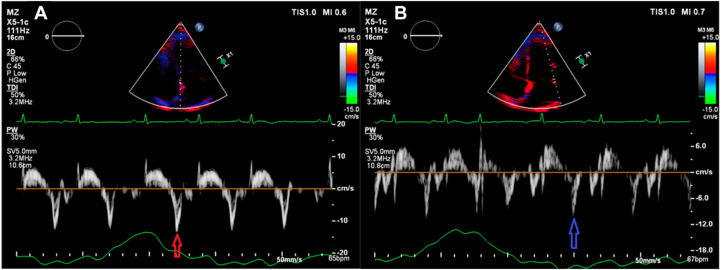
Case 3. Two-dimensional TTE, apical 4-chamber view (A, B) showing very high (supranormal) septal e’ (*red arrow*); annulus paradoxus and lower lateral e’ (*blue arrow*) vs higher septal e’; and annulus reversus.
